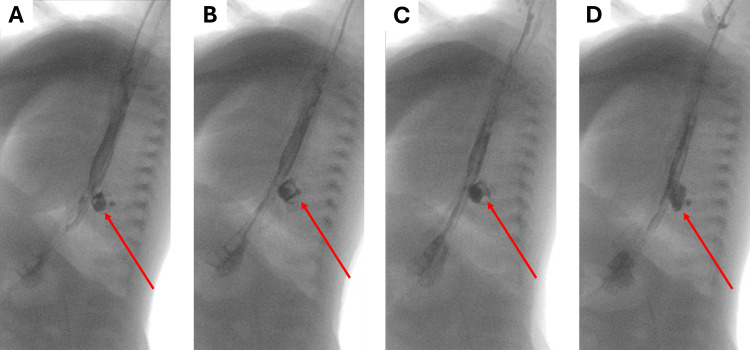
The radiocontrast evaluation shows signs of anastomotic leakage one week after the final surgery. (A) Initial X-ray taken upon an introduction of the contrast medium into the esophagus; (B) second X-ray taken after a waiting period; (C) third X-ray taken after a waiting period; (D) fourth X-ray taken after a waiting period. The time difference between each panel was at the discretion of the radiographer performing the evaluation. The red arrows point to the signs of radiocontrast leakage from the site of the esophageal anastomosis.
